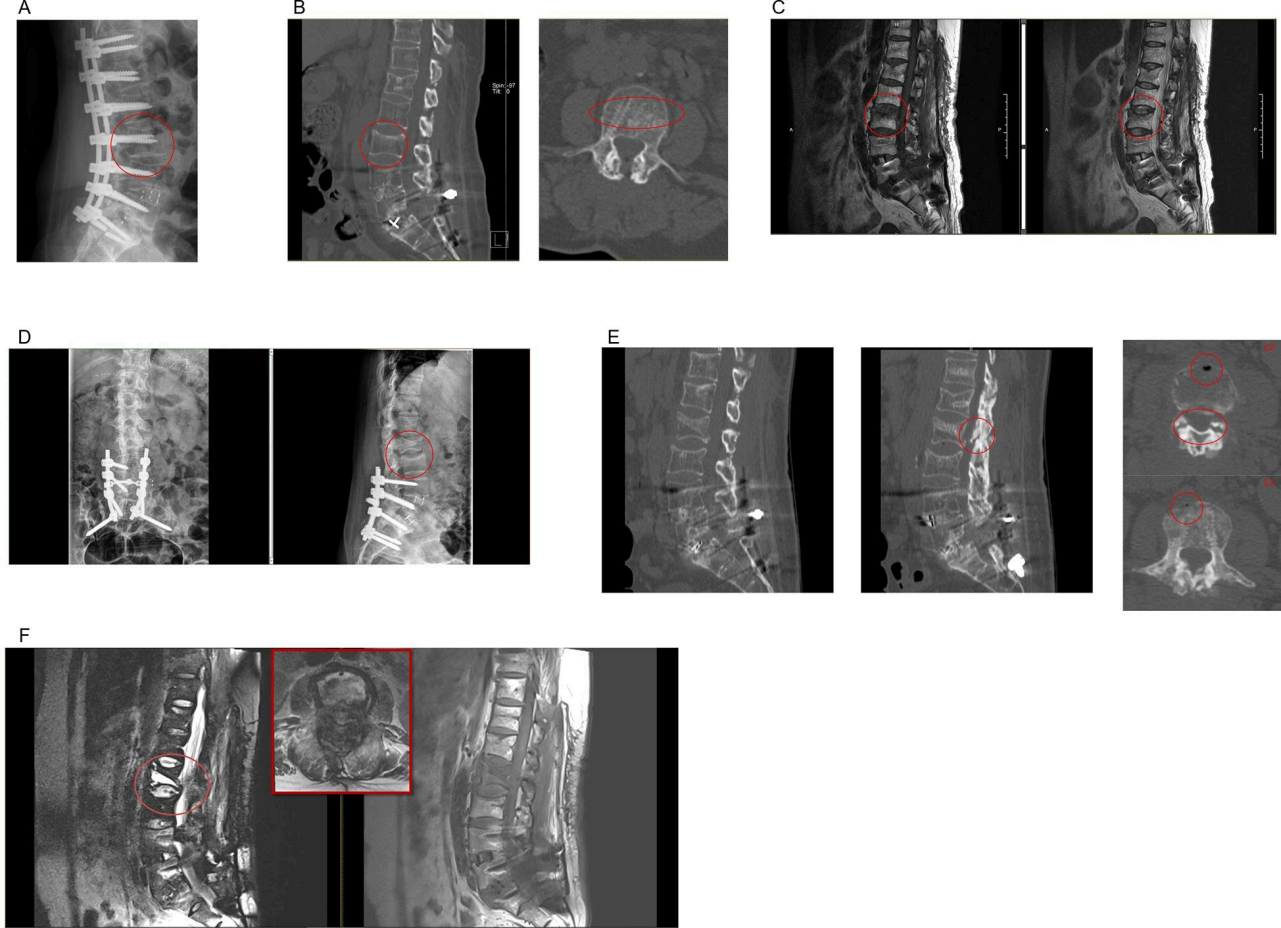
(A,B) Radiograph (A) and corresponding sagittal (left) and transverse (right) CT images (B) showing the same vertebrae marked with a red circle. The fracture line is marked with a red oval. (C) Magnetic resonance images (MRI) image 3 months post-operatively showing cauda equina damage. Red circles highlight the mild fracture with relatively preserved vertebral shape. (D) Posterolateral radiograph 4 months post-operatively, showing a crater-like osteoporotic lesion (red circle) in both L2 and L3, with more severe involvement in L2. (E) Sagittal (left) and transverse (right) CT images showing vacuum clefts on L2 and L3 (red circles), an osteophyte protruding into the spinal canal, and L2 and L3 fractures. (F) MRI showing spinal cord compression (red circle), with the severity evident in the transverse section (red rectangle).
